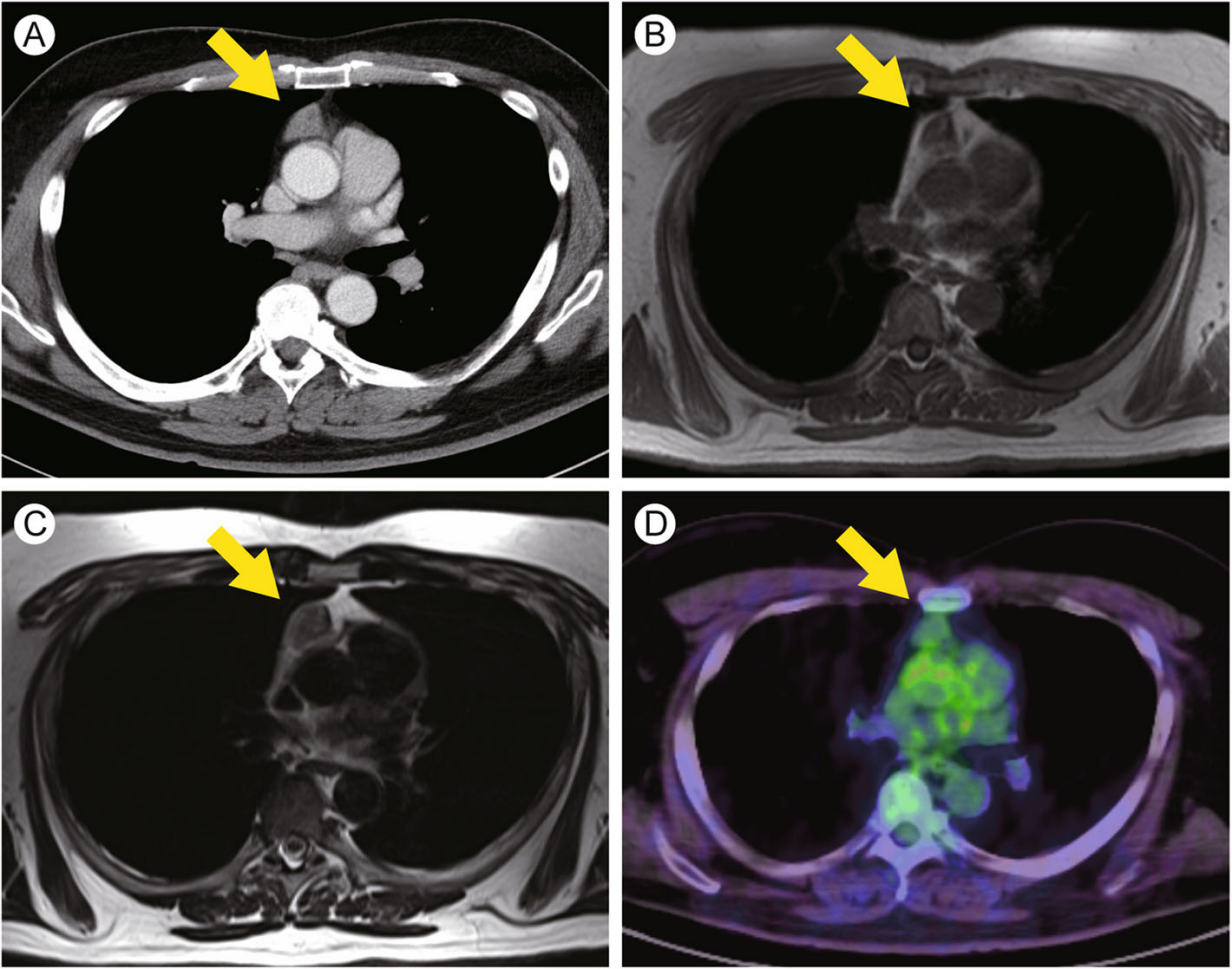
Computed tomography (CT), magnetic resonance imaging (MRI), and positron emission tomography (PET)/CT images of the mediastinal mass (yellow arrow). a An enhanced CT scan shows a weakly enhanced mass (size, 6.6 × 2.4 × 2.2 cm). The ratio of the mediastinal mass to the heart was 0.19. b and c Magnetic resonance imaging T1-weighted (b) and T2-weighted (c) images reveal areas of isodensity intermingled with high-density areas. d PET/CT images demonstrate weak fluorodeoxyglucose uptake
